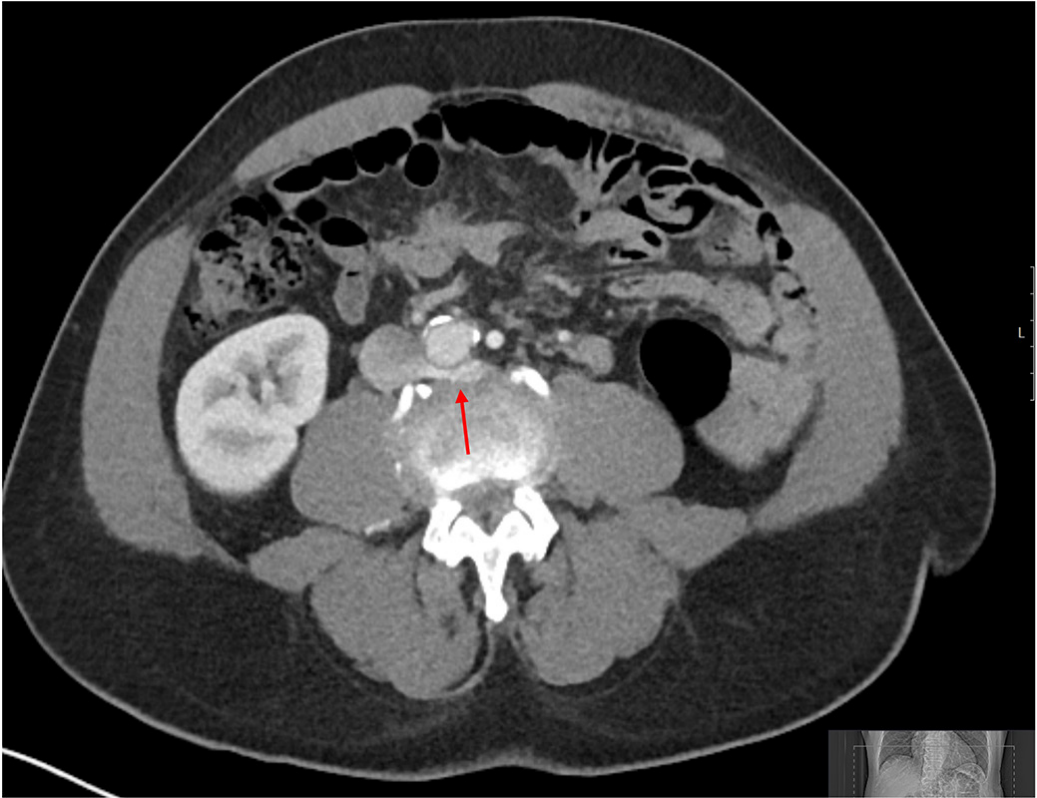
Axial image of portovenous CT at the level of L3/4. Red arrow demonstrating the left upper pole renal vein branch taking a retroaortic course with mild compression.
